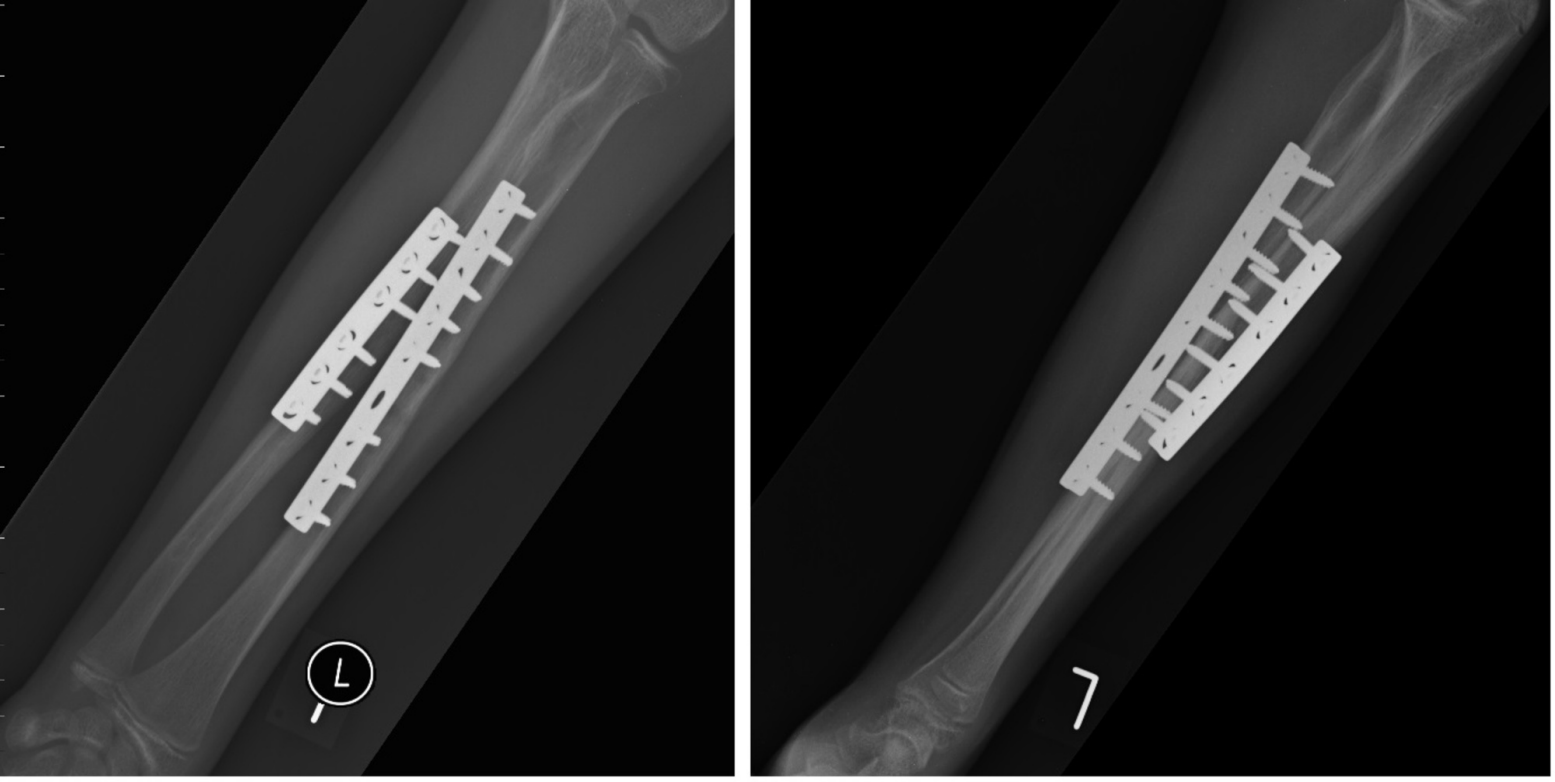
Postoperative radiographs Orthogonal radiographs of the forearm at two month follow-up visit showing union of both bones with no evidence of synostosis.
